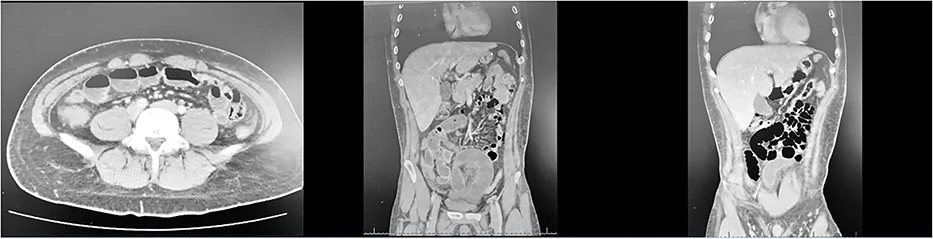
CT abdomen with IV contrast showed a dilated small bowel loop with an air–fluid level of 3.6 cm, a transitional zone in the distal ileum, and a collapsed large bowel loop, without any signs of bowel ischemia.
